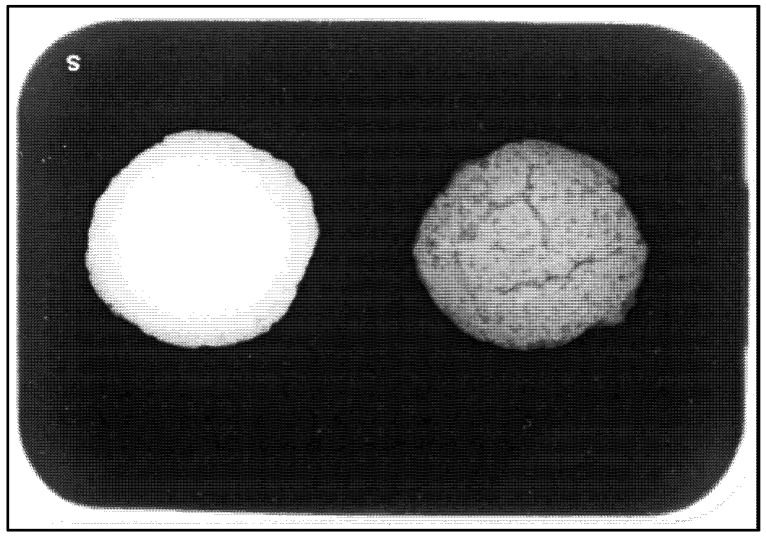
Radiograph of (left) Sample 4 experimental pulp-capping agent and (right) MTA.
